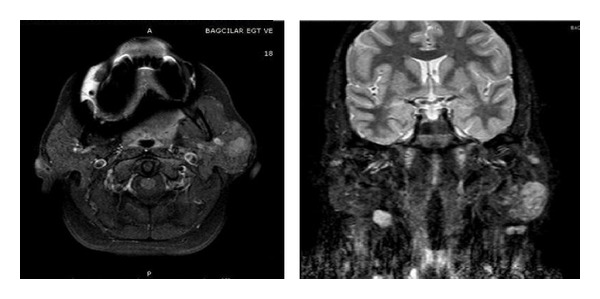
MRI demonstrates solid mass lesion in left parotid gland. Fat-suppressed T2-weighted images show mild hyperintensity, T1-weighted images show hypointensity, and postcontrast images show low contrast enhancement.
